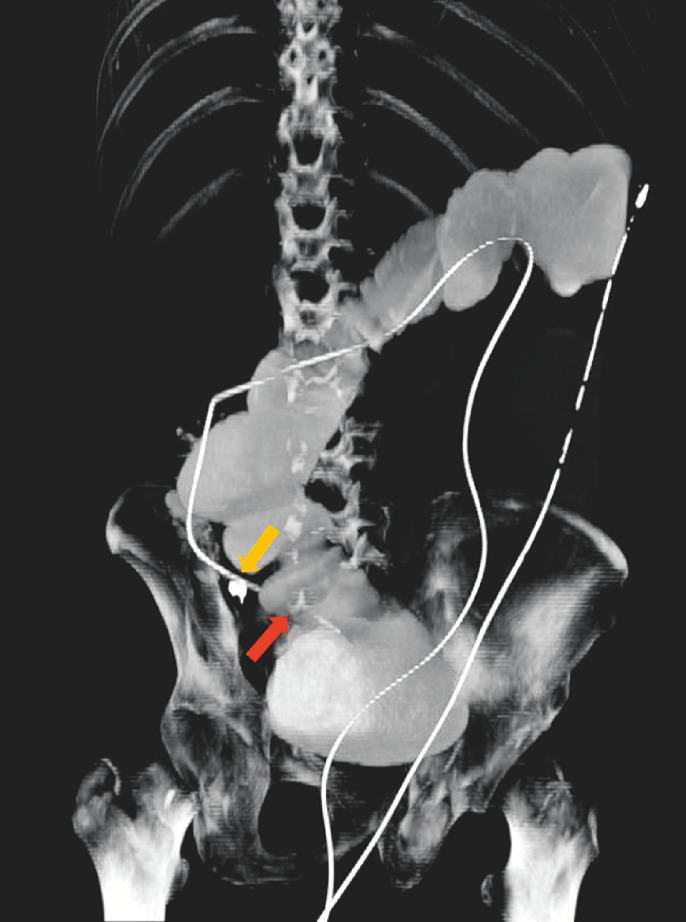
Computed tomography (CT). Approximately 200 mL of contrast agent was injected into the intestine through the colonic transendoscopic enteral tubing (TET) tube. Following a 10-minute ambulation, CT was conducted and displayed the entire TET tube, dilated distal ileum, and a segment of the proximal colon. The yellow arrow indicates clips fixed onto the intestinal wall. The red arrow highlights the TET tube passing through the stricture into the terminal ileum.
